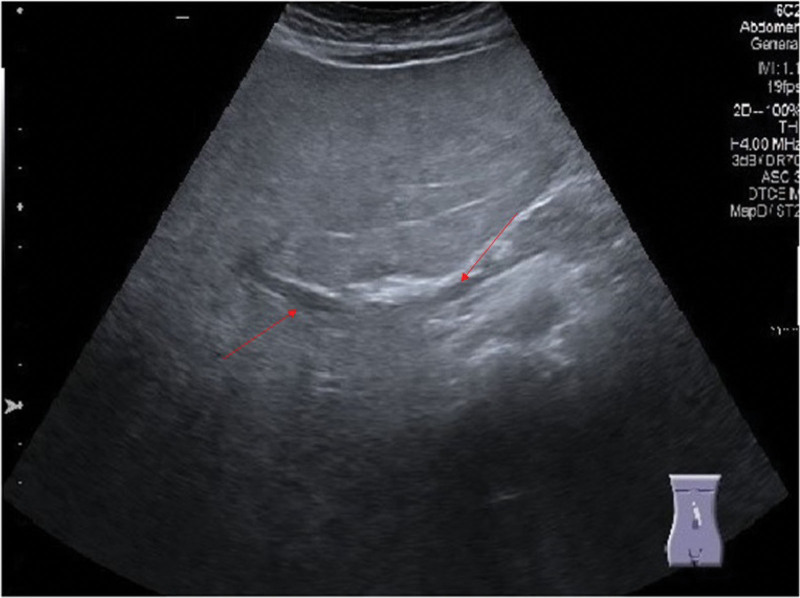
Abdominal ultrasound. Routine checkup. Status after cholecystectomy, surrounding area, liver parenchyma with lower echogenicity (red arrows). In addition, the liver was visible fragmentarily, enlarged, with increased echogenicity of the parenchyma. Intrahepatic bile ducts did not dilated. The pancreas in the area of the head was not enlarged, discreetly heterogeneous, otherwise obscured.
